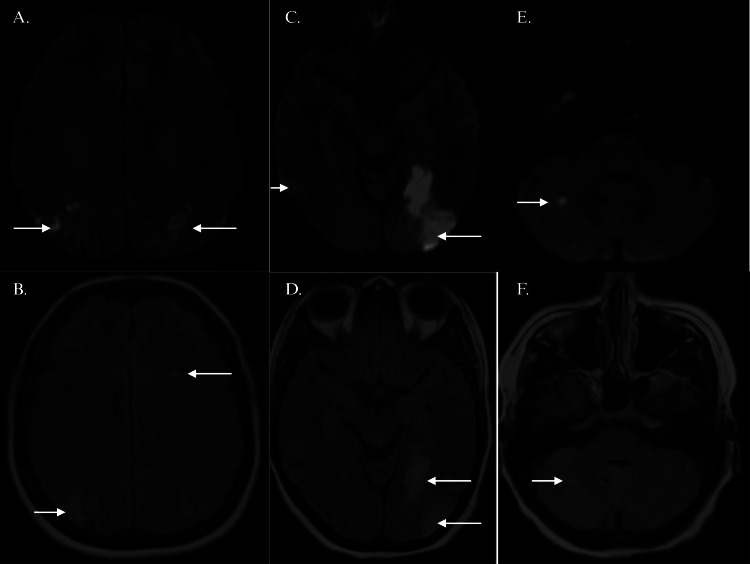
MRI brain showing ischemic infarcts (white arrows) in (A, B) bilateral frontoparietal lobes, (C, D) posterior cerebral artery territory, and (E, F) right cerebellum MRI: magnetic resonance imaging
